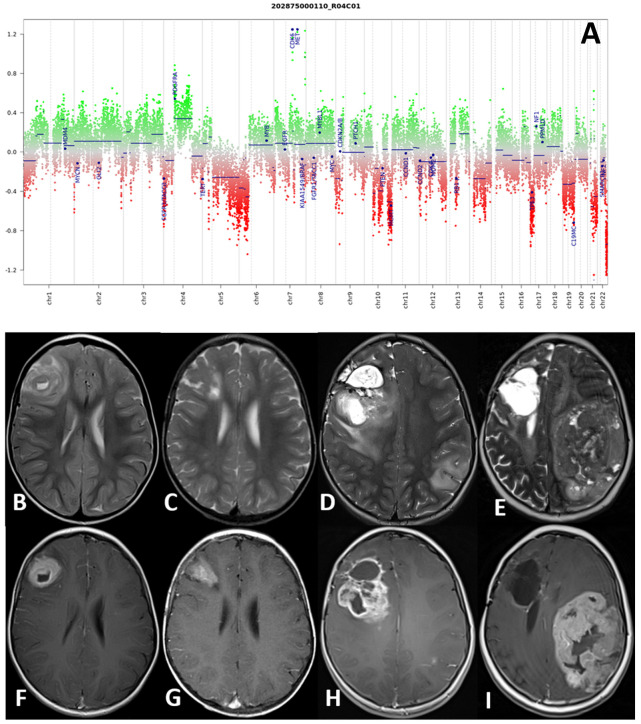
(A–I) Molecular profile (A) and MRI at four consecutive time-points of patient number 4. DNA methylation profile showed a complex copy number variation (CNV) with CDK6 and MET amplification, and a platelet-derived growth factor A (PDGFRA) gain (A). Axial TSE T2-weighted (upper row) and post-gadolinium SE T1-weighted (lower row) sequences at four consecutive time points: at presentation (B,F), at first local recurrence after surgery (C,G), at second local recurrence after reoperation (D,H), and spreading to the contralateral hemisphere (E,I). The right frontal high-grade glioma and recurrences present with a non-homogeneous hyperintense signal on T2-weighted and intense and non-homogenous contrast enhancement on T1-weighted images.
